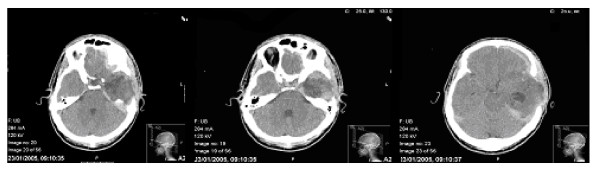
Postoperative computerized tomography(CT) scan. A follow-up CT scan 9 months later shows a modified decompressive craniotomy for removal of hematoma.
